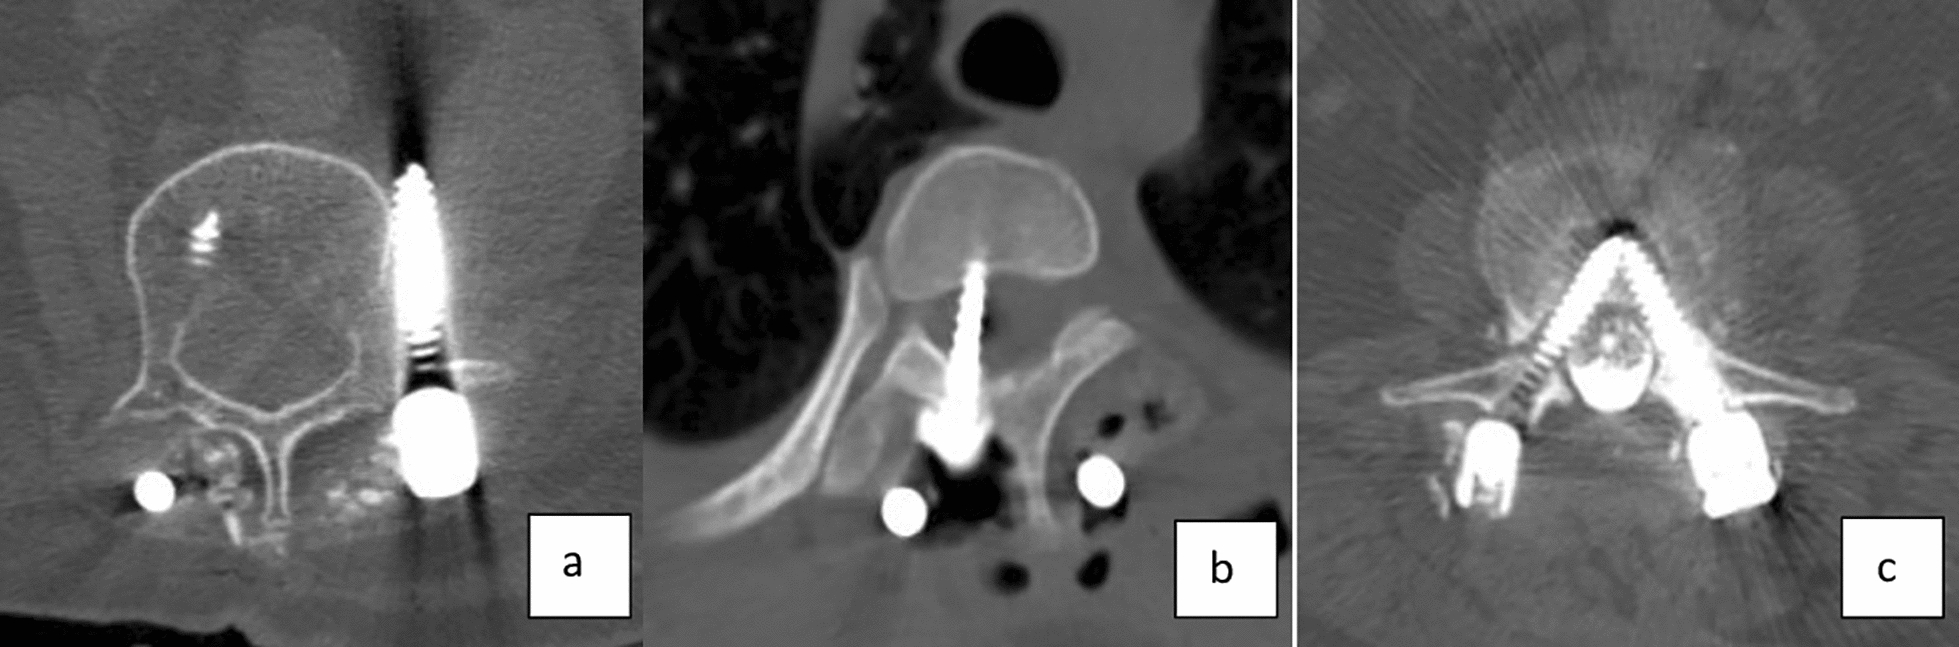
a–c Axial CT scans demonstrating screw malposition
